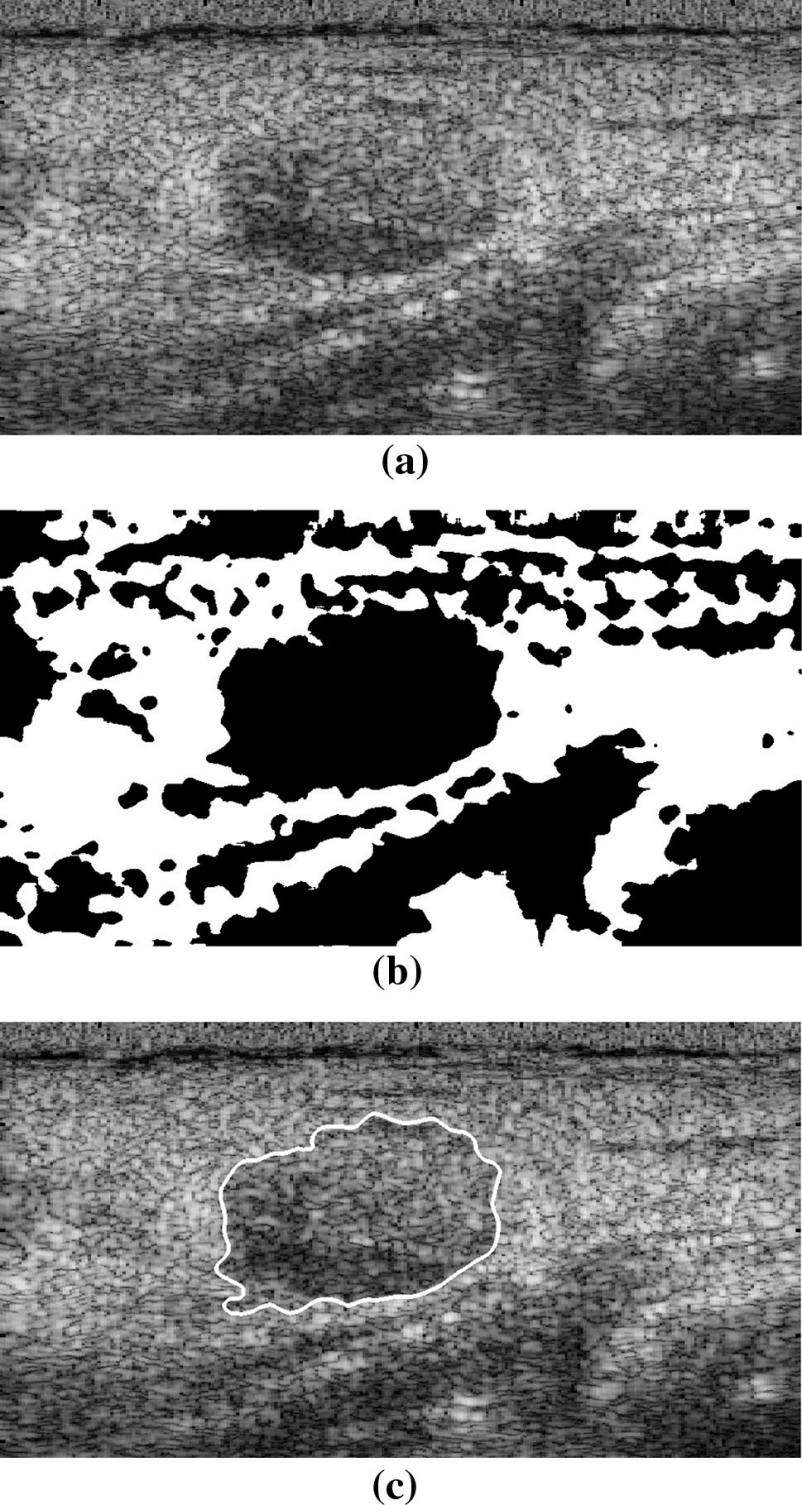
a Breast ultrasound image. b Image after adaptive thresholding. c Tumor contour extracted using DE
